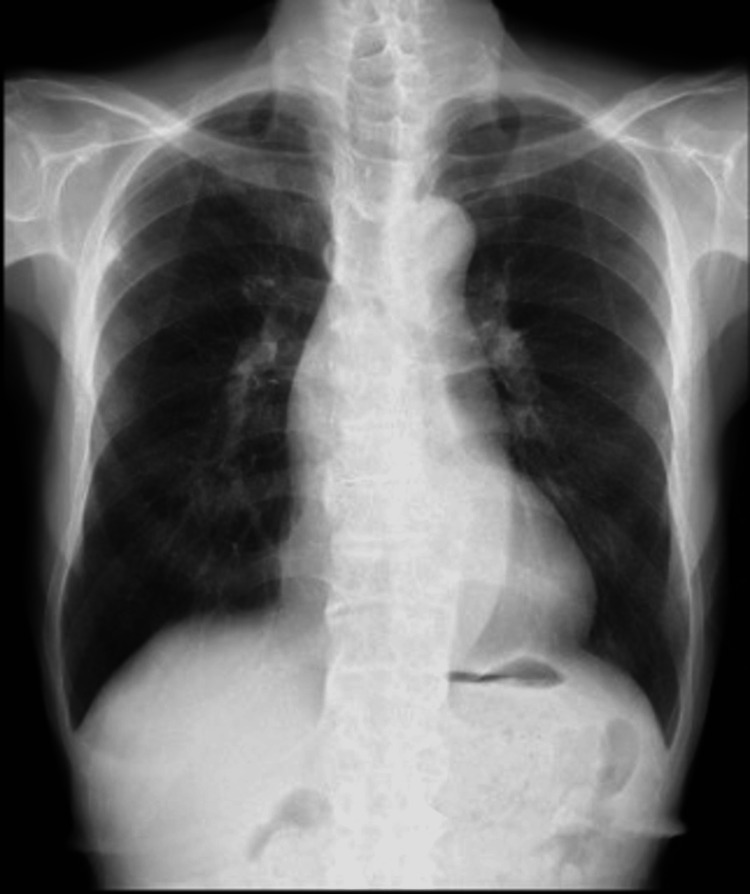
Preoperative chest radiograph. Clear lung fields with a cardiothoracic ratio of 48.0% were observed in the upright position.
